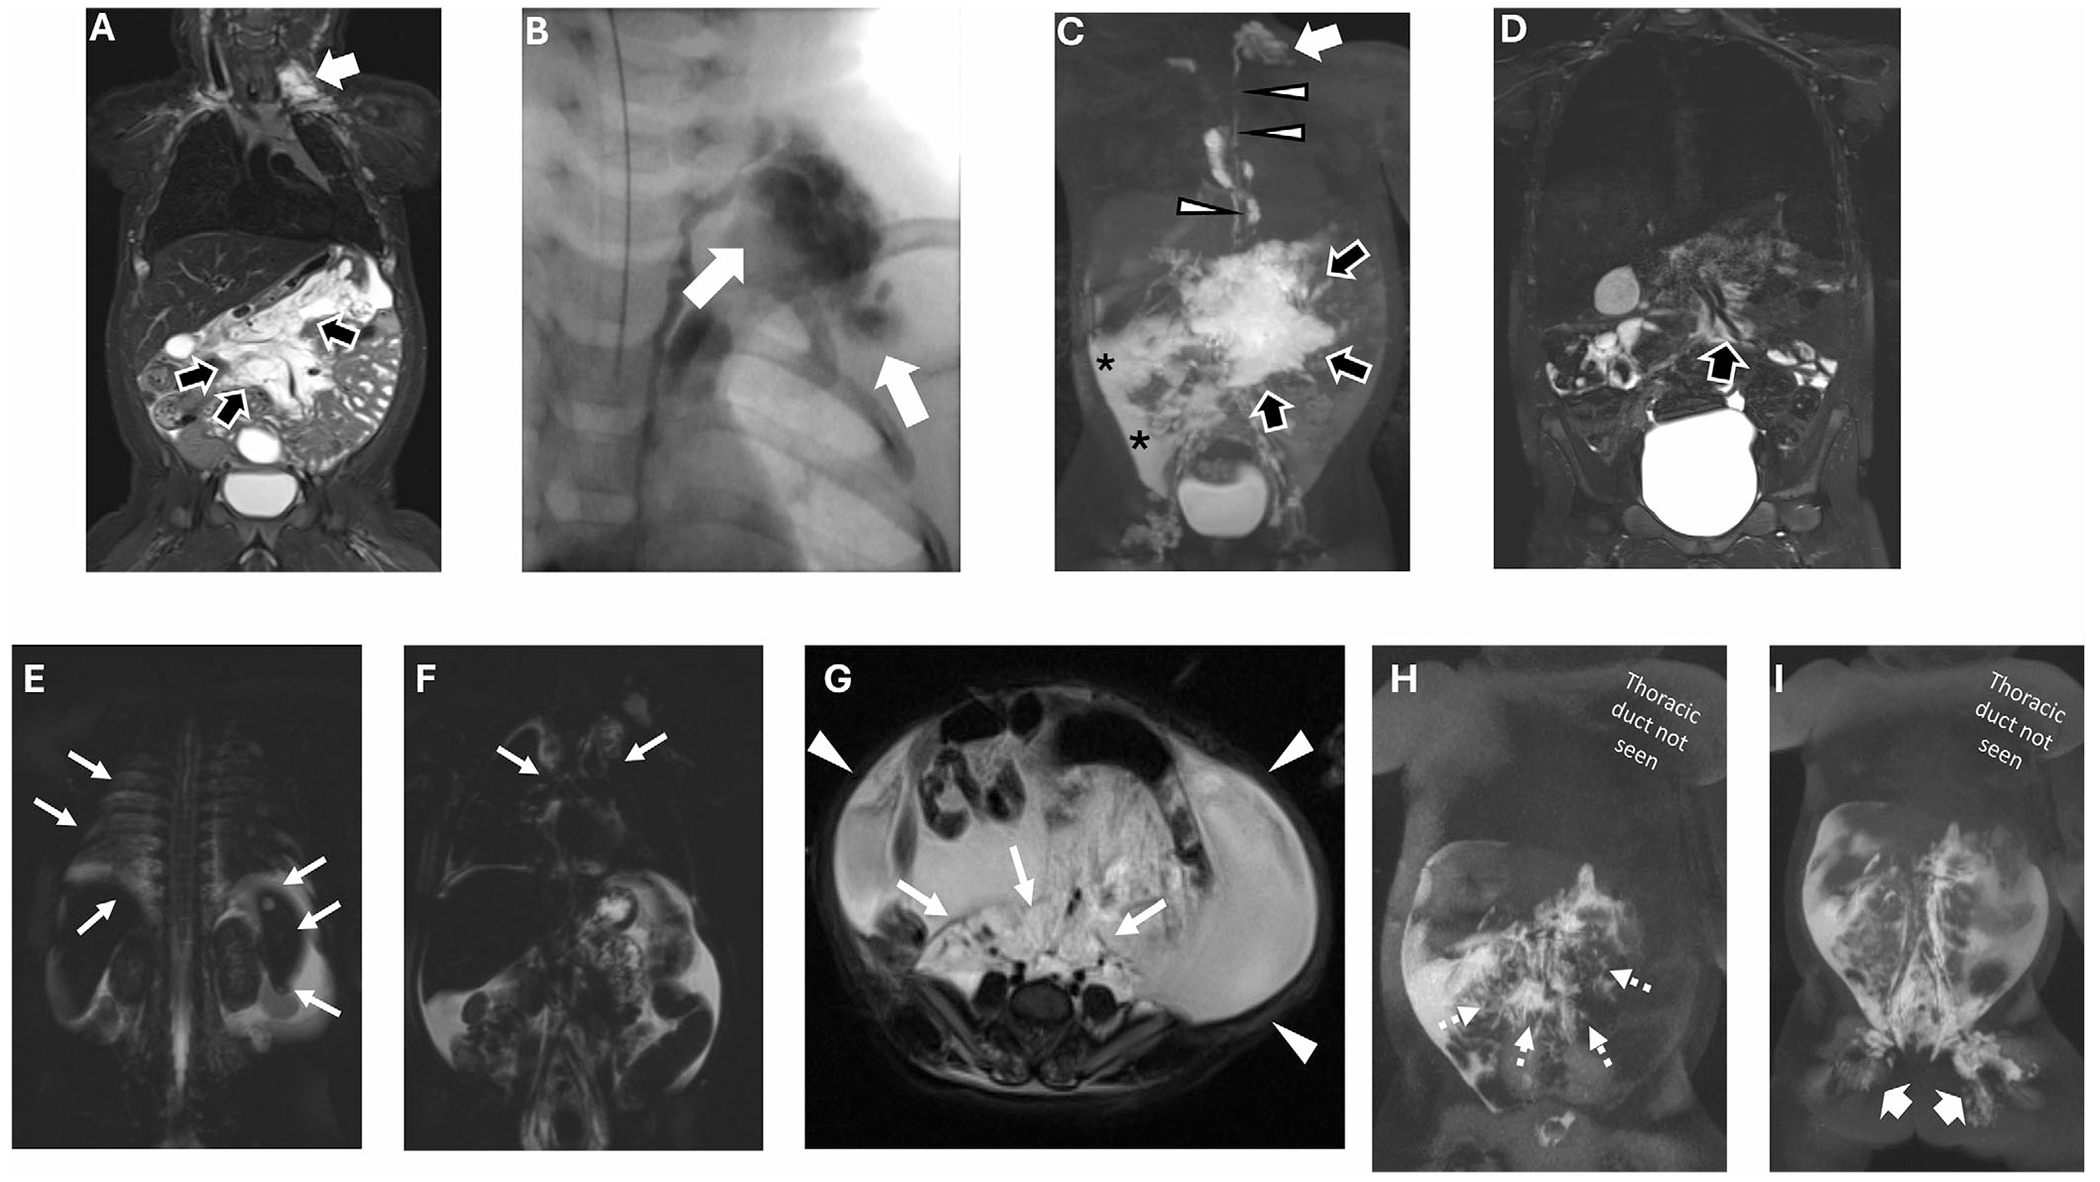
Imaging for Cases 5 and 6. (A–D) Patient 5: 4-year-old with generalized lymphatic anomaly (GLA) and central conducting lymphatic anomaly (CCLA). (A) Magnetic resonance imaging (MRI) during infancy shows lymphatic malformation centered in the mesentery (black arrows), with a smaller lymphatic malformation in the left supraclavicular region (white arrow). (B) Fluoroscopic imaging from direct lymphangiography after antegrade trans-abdominal access of the thoracic duct showing direct connection of the thoracic duct with the supraclavicular lymphatic malformation (white arrows). (C) Magnetic resonance lymphangiography (MRL) demonstrates the abdominal lymphatic malformation (black arrows) from liver and mesenteric injection with peritoneal leak (asterisks), as well as supraclavicular lymphatic malformation (white arrow) connected to the thoracic duct (white arrowheads) on inguinal injection. (D) Follow-up MRI at age 4 years after initiation of sirolimus showing a significant decrease in malformations (black arrow). (E–I) Patient 6: 14-month-old with GLA and lymphatic malformation (LM). (E–G) Axial and coronal T2-weighted images with fat suppression during infancy show infiltrative lymphatic malformation in the mediastinum, pleura, spleen, retroperitoneum, and pelvis (white arrows) and ascites (white arrowheads). (H and I) Maximum-intensity projection images of T1-weighted spoiled gradient echo images after injection of contrast into lymphatics show retrograde flow of contrast into mesentery and retroperitoneum (dashed arrow) after liver and mesenteric injection, but not into the thoracic duct. After groin injection, there is similar filling of retroperitoneal lymphatics and abnormal retrograde flow (thick arrows), but the thoracic duct still does not fill with contrast.
